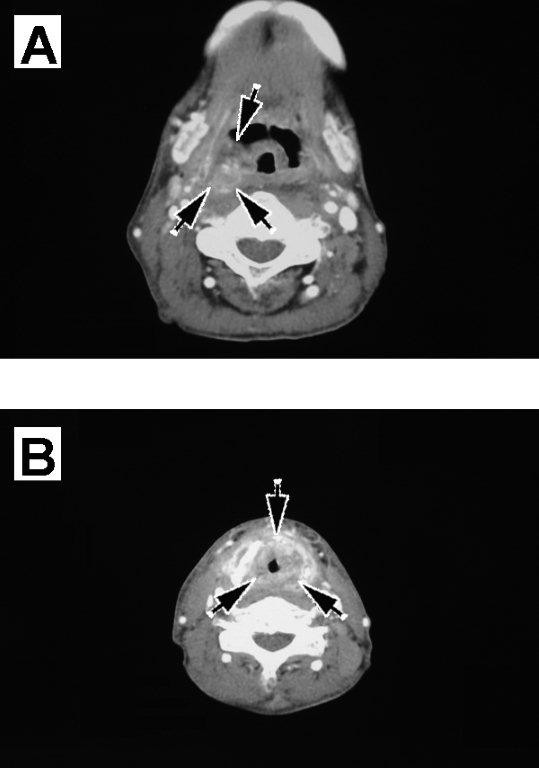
Follow-up CT results. (a) A suggestive space-occupying lesion in the right lateral pharyngeal wall at the level of the epiglottis, and (b) a suggestive circumferential space-occupying lesion in the mucosa and airway constriction at the level of the thyroid cartilage.
